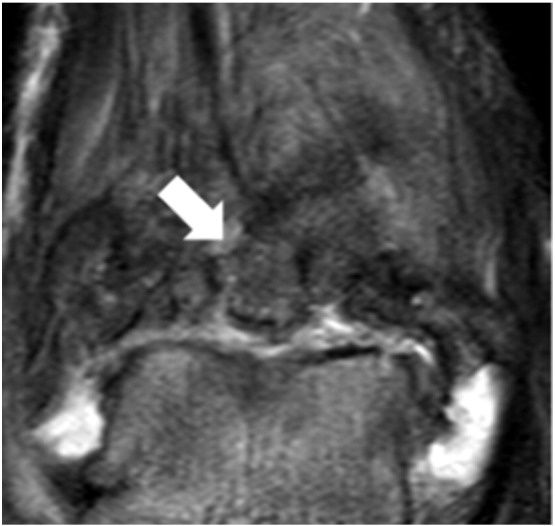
T2-weighted MRI showed an area of heterogeneous intensity (arrow).
